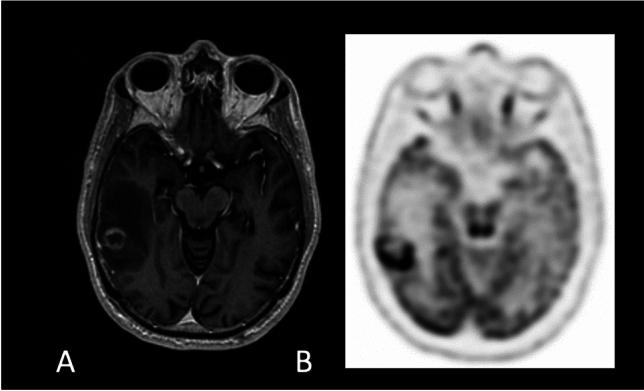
Example of hypermetabolism in EBV-associated brain lymphoma. A Ring-enhancement lesion on contrast-enhanced T1-weighted sequences; B Hypermetabolism on PET-CT
